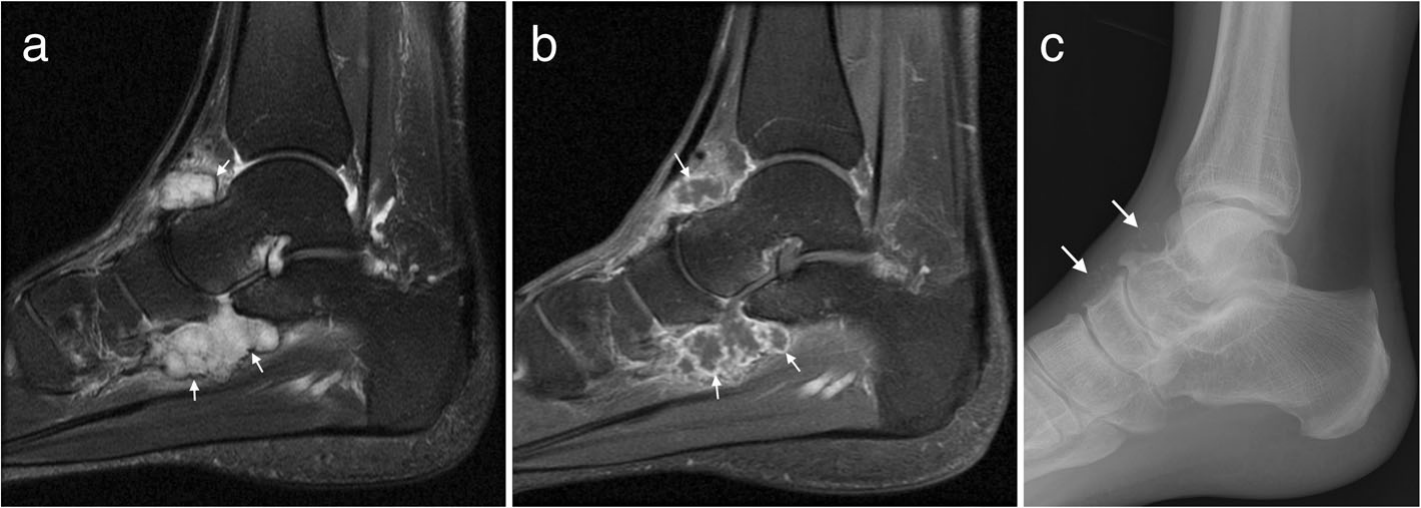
Synovial chondromatosis. a Sagittal T2FS image of the ankle demonstrating a lobulated T2 hyperintense lesion with a low signal rim (arrows) and b avid synovial enhancement on post contrast T1FS imaging (arrows). c Mineralisation is better appreciated on the corresponding radiograph (arrows).
